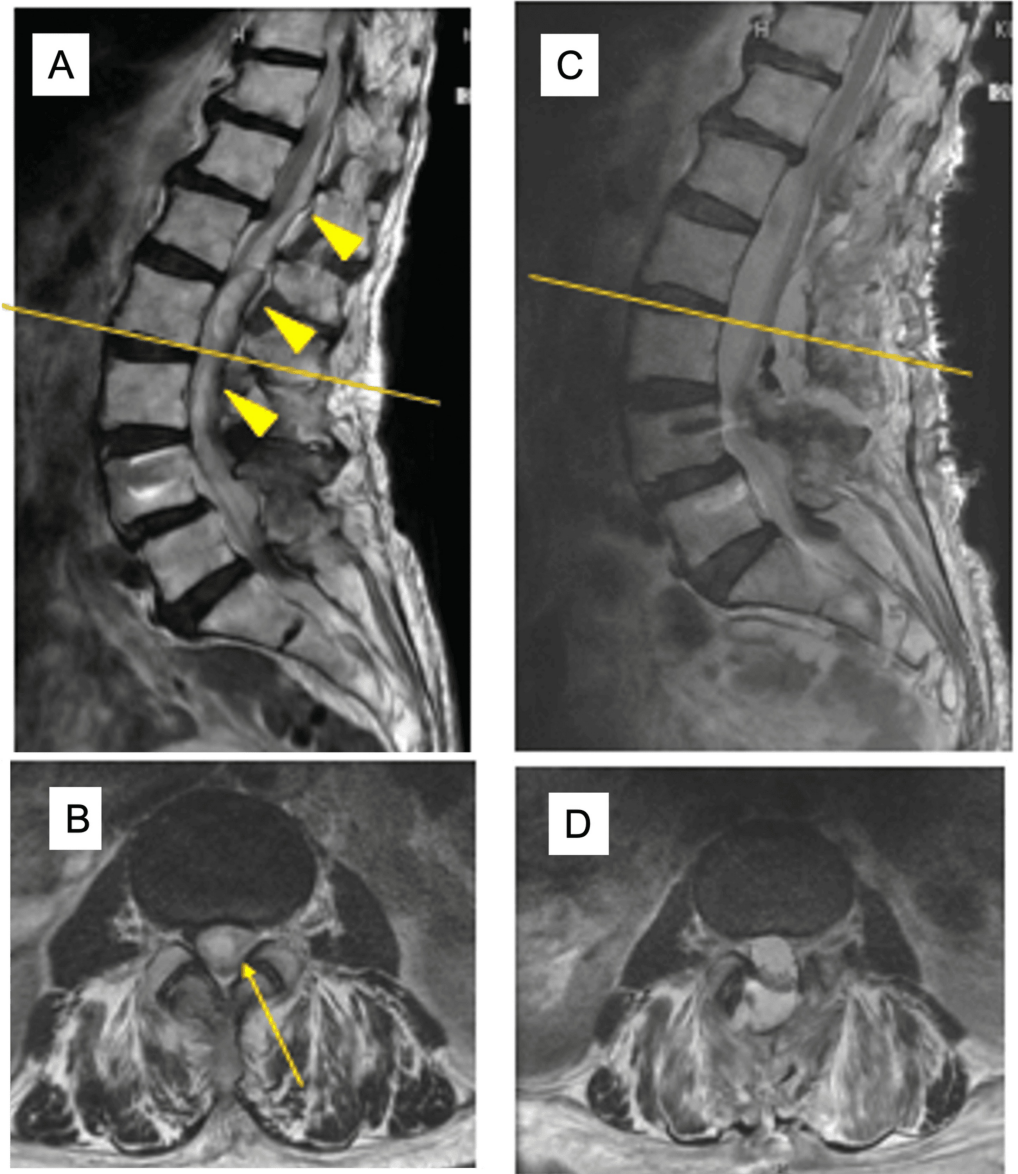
Pre- and postoperative MRI findings in Case 2. (A) Preoperative sagittal T2-weighted MRI demonstrating a hyperintense dorsal epidural mass extending from L1 to L4 (yellow arrowhead). (B) Preoperative axial T2-weighted MRI showing compression of the dural sac by epidural hematoma (yellow arrow). (C) Postoperative sagittal T2-weighted MRI confirming adequate decompression of the spinal canal. (D) Postoperative axial T2-weighted MRI revealing complete evacuation of the hematoma and decompression of neural elements MRI: magnetic resonance imaging
